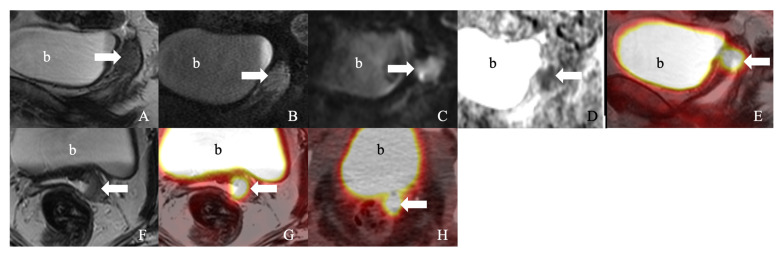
A 55-year-old woman with squamous cell carcinoma of the cervix, status post hysterectomy. (A). Sagittal T2-weighted imaging (T2WI), (B). post-contrast sagittal T1-weighted imaging (T1WI), (C). coronal diffusion-weighted image (DWI), (D). coronal apparent diffusion coefficient (ADC), (E). coronal fused T2WI, and (F). axial T2WI. (G). Axial fused T2WI positron emission tomography/MRI showed an enhancing (18)F-fluorodeoxyglucose (FDG) avid plaque-like thickening at the left cervix (arrow) with restricted diffusion. (H). An axial positron emission tomography/computed tomography image showed ill-defined FDG avidity (arrow). b: urinary bladder.
